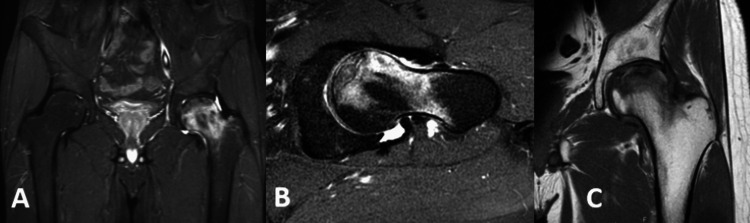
MRI pelvis A: FLAIR pelvis coronal view showing severe marrow edema of the left femoral head. B: Left hip axial view showing diffuse marrow edema, early subchondral collapse, and resultant joint effusion. C: Left hip T1 coronal view showing diffuse marrow edema with early subchondral collapse. FLAIR: Fluid attenuated inversion recovery
